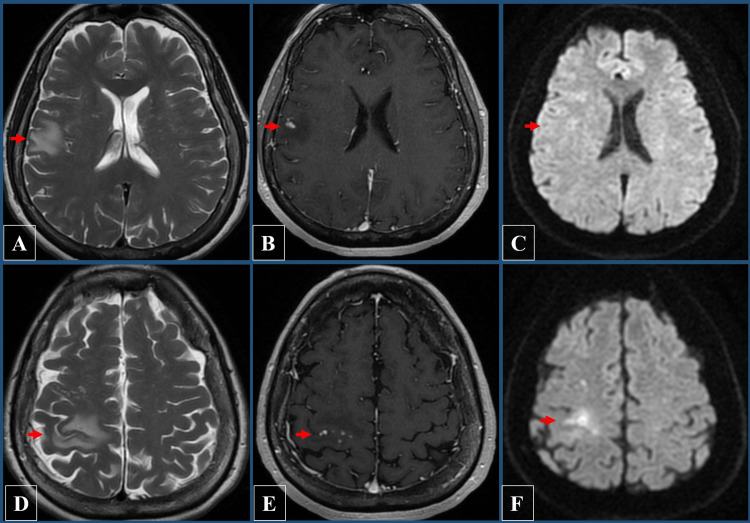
Magnetic resonance imaging (MRI) demonstrating axial images at three-month follow-up. (A, D) T2 fluid-attenuated inversion recovery (FLAIR) images showing lesions with peri-lesional edema in the right hemisphere (red arrows). (B, E) T1-weighted post-contrast images demonstrating annular enhancing lesions, consistent with non-ischemic cerebral enhancing (NICE) lesions (red arrows). (C, F) Diffusion-weighted imaging (DWI) showing the lesions with restricted diffusion (red arrows).
